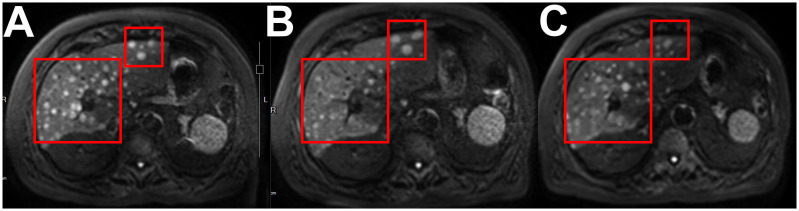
MRI images showing tumour response to pembrolizumab plus bevacizumab. Axial contrast-enhanced T2-weighted MRI images at three time points during treatment with reduced-dose pembrolizumab plus bevacizumab. Due to the diffuse, multifocal nature of the intrahepatic disease—with numerous small nodules distributed across both lobes—representative images are shown rather than individual measured target lesions, as the latter would not have been reliably selectable or reproducible per RECIST 1.1 criteria. The lesions appear as hyperintense foci (white) on T2-weighted imaging, corresponding to areas of viable tumour, which were confirmed by arterial phase hyperenhancement on dynamic contrast-enhanced sequences. (A) October 2023 (pre-treatment): Baseline imaging before initiation of pembrolizumab-bevacizumab, showing multifocal intrahepatic progression with hyperintense lesions within the red boxes. (B) January 2024 (after 3 cycles): Follow-up imaging after three cycles of combination therapy, demonstrating partial response (PR) according to RECIST 1.1 criteria, with significant reduction in size and signal intensity of the lesions. (C) January 2025 (15 months): Imaging after 15 months of continuous treatment, showing sustained response with no evidence of new lesions or progression.
